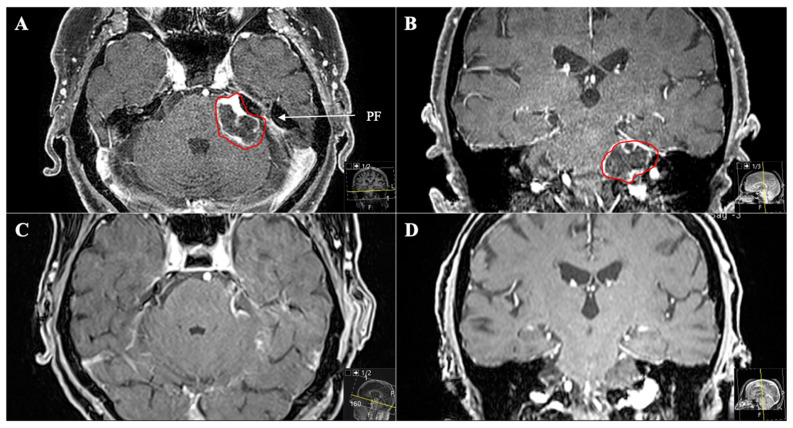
A 53-year-old female patient presented with vomiting, slight ataxia, facial pain and trigeminal hypesthesia (V1,V2) for three months. (A) Preoperative axial and (B) coronal T1-weighted gadolinium-enhanced MRI, showing a space-occupying cystic trigeminal schwannoma invading the cerebellopontine angle (Samii Type B). (C) Postoperative axial and (D) coronal MRI control, indicating complete resection via a retrosigmoid approach. Postoperatively, new temporary slight hypoacusis and facial neve palsy (House and Brackmann Grade III) occurred.
